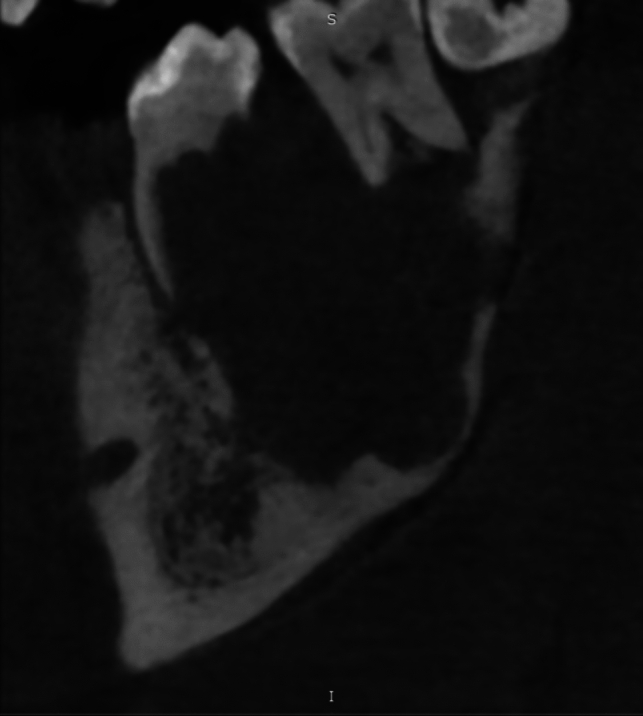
CT scan shows the osteolytic lesion and the erosion of the dental elements. In this image, the ragged aspect of root resorption is particularly evident
